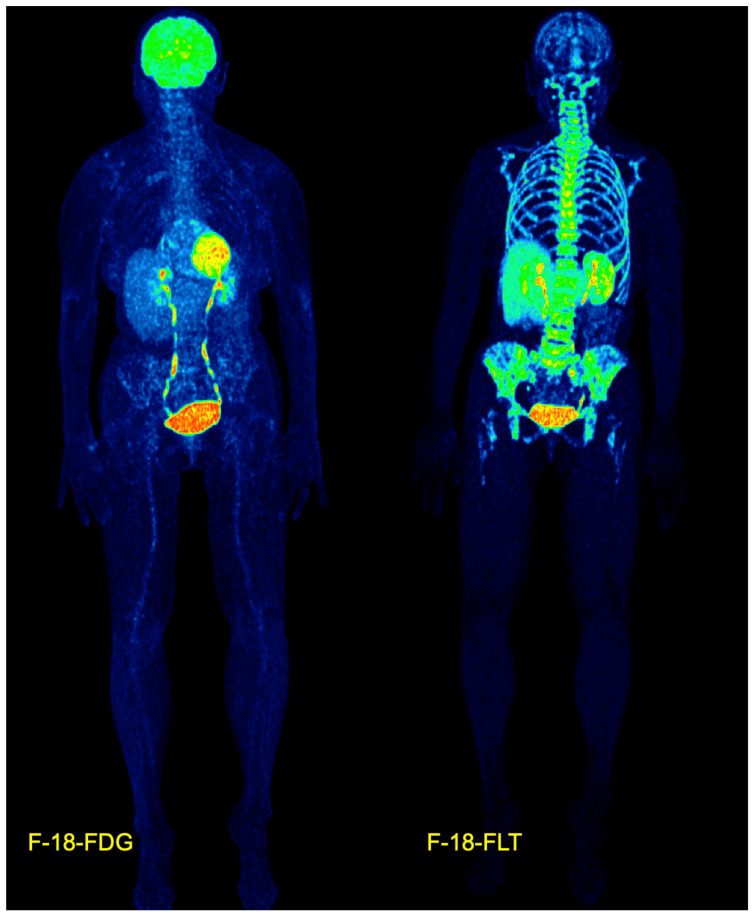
Maximum intensity projection (MIP) images of a patient with multiple myeloma 1 h after i.v. [^18^F]FDG injection (left side) and 1 h after [^18^F]FLT injection (right side). The [^18^F]FLT images show high uptake in the bone marrow of the axial skeleton, the pelvic bones, the spleen, and the liver. The [^18^F]FDG images show no focal or diffuse enhanced uptake in the bone marrow. Normal [^18^F]FDG excretion in the urinary tract.
